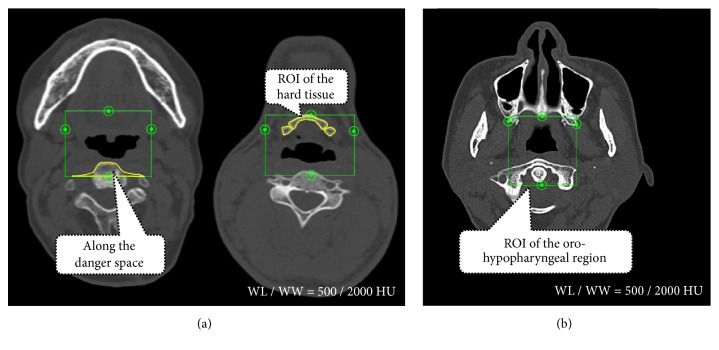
The region of interest (ROI) used for calculating the hard tissue and oro-hypopharyngeal region volumes. The ROI of the hard tissue and oro-hypopharyngeal region were set by manually tracing all the axial cross-sectional images of the oro-hypopharyngeal region. To set the ROI, a danger space was used in the area of the cervical vertebrae to maintain bone morphology continuity. All the hard tissue and oro-hypopharyngeal region ROIs in the oro-hypopharyngeal region assessed using multislice computed tomography data at baseline and data with the mandibular advancement appliance (MAA) were superimposed. Abbreviations: WL/WW, window level/window width.
